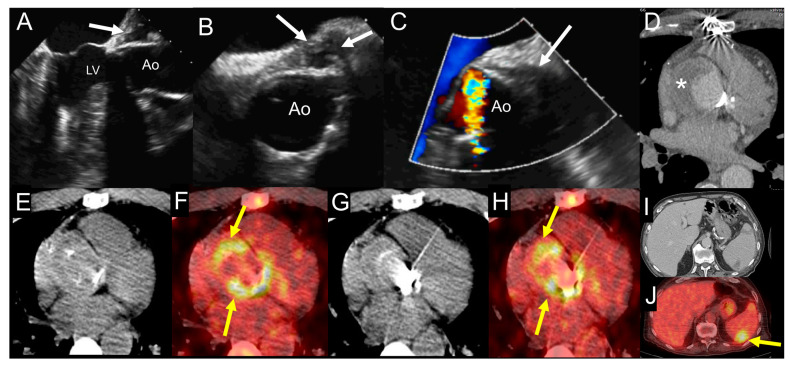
Example of the use of multimodality imaging. A patient with history of aortic valve replacement with mechanical prosthesis and ascending aorta graft presented four years later with acute right lower limb ischemia due to occlusion of proximal fibular, anterior tibial, and posterior tibial arteries treated with revascularization attempts and finally leg amputation. During hospitalization, the patient had fever with increased erythrocyte sedimentation rate and C-reactive protein. TTE (A) and at TOE (B,C) show the presence of hyperechogenic periprosthetic area (white arrows), most likely consistent with abscess. Blood culture was negative. The fluoro-18-fluorodeoxyglucose positron emission tomography/computed tomography ((^18^F)FDG PET/CT) exam including CCTA (D-J) was performed showing an organized fluid perigraft collection surrounded by thick walls (asterisk) that enhance after iodinated contrast injection on CCTA images (D), which is associated to intense uptake of (^18^F)FDG around the aortic valve prosthesis, as shown by the yellow arrows ((E,G) show noncontrast CT transaxial images while (F,H) show the fused PET/CT images). Myocardial suppression of (^18^F)FDG uptake is achieved by high-fat, low-carb diet. In addition, the whole-body images showed an area of spleen uptake, consistent with septic embolism ((I,J), noncontrast CT and fused PET/CT transaxial images, respectively), as indicated by the yellow arrow. Ao: ascending aorta; LV: left ventricle.
